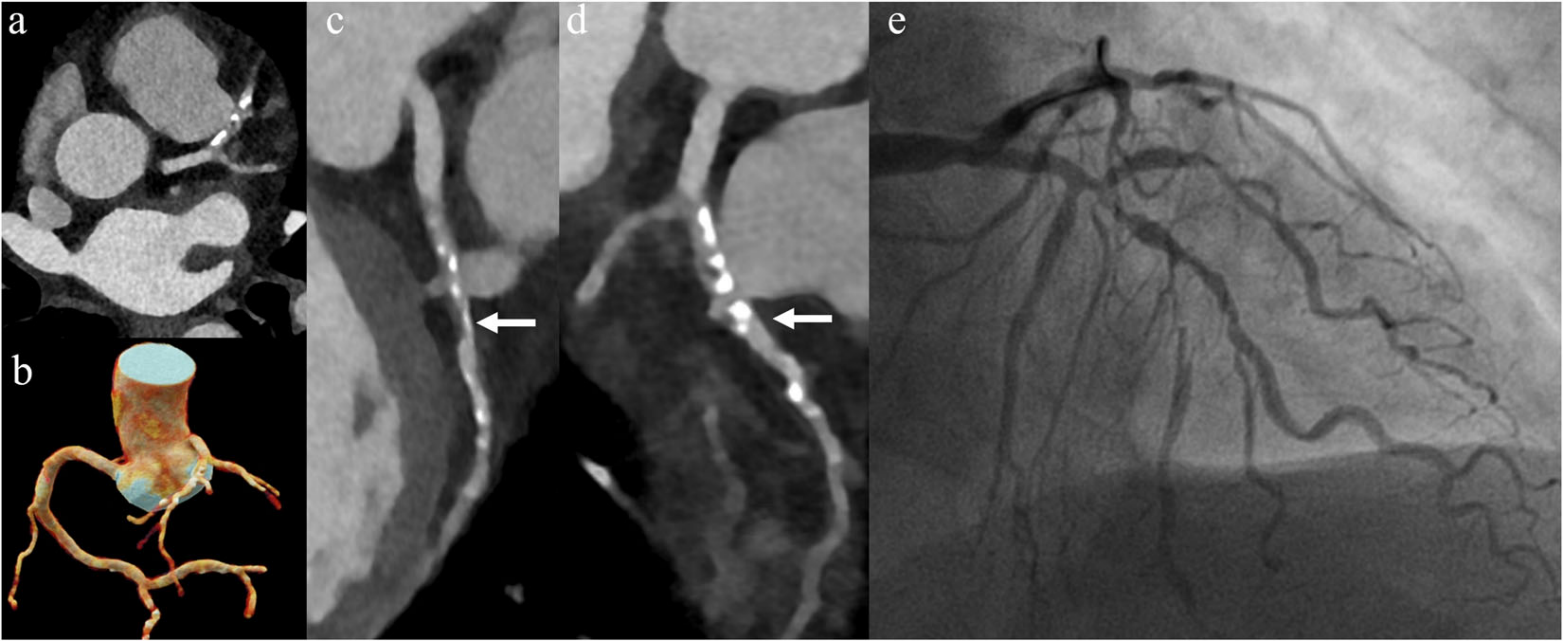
CCTA in a 74-year-old Female (HR = 75 bpm, BMI = 18.3 kg/m^2^, Contrast agent flow = 1.5 mL/s, Contrast agent volume = 15 mL, CAD-RADS = 4) with chest pain for 1 day. a shows CT value of AAO was 362 HU. b shows the coronary arteries, cine volume rendering technology images. c, d The anterior descending artery representative curved multiplanar reformation shows the severe stenosis and diffuse calcification in the lumen (White arrow). e Percutaneous coronary angiography shows severe stenosis of the left anterior descending artery
